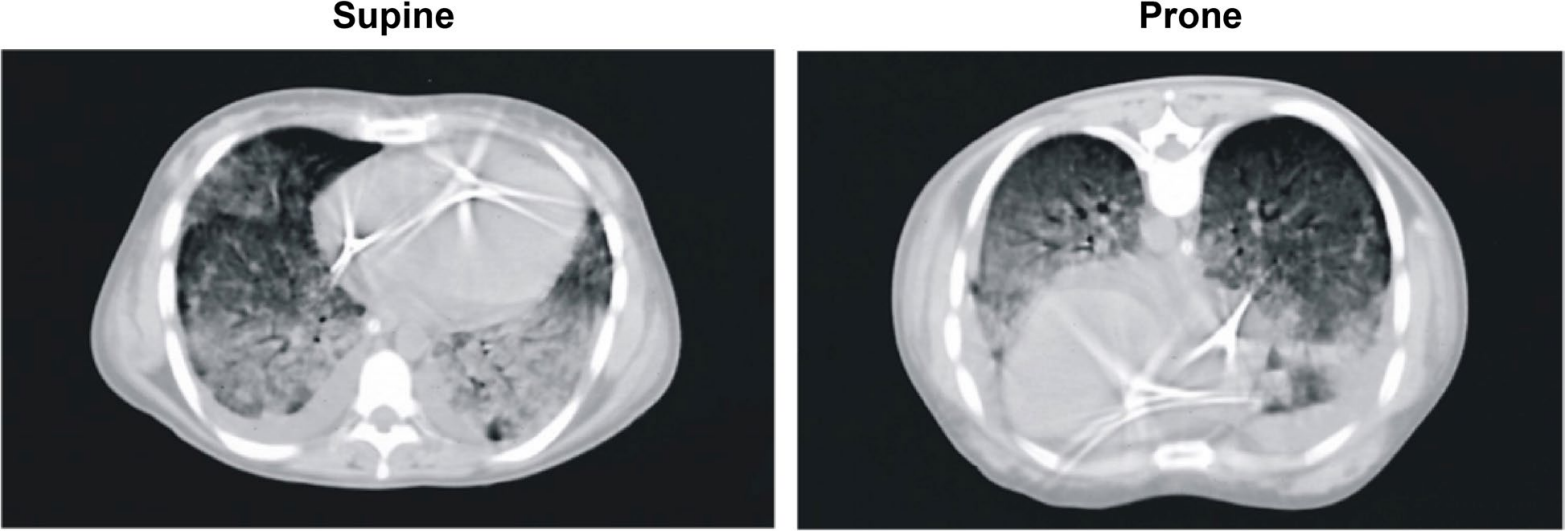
CT-scans in supine *vs.* prone position at end-expiration. Representative CT-scans of a patient with ARDS, showing that lung densities redistribute from the dorsal to ventral regions when moving from supine to prone position
